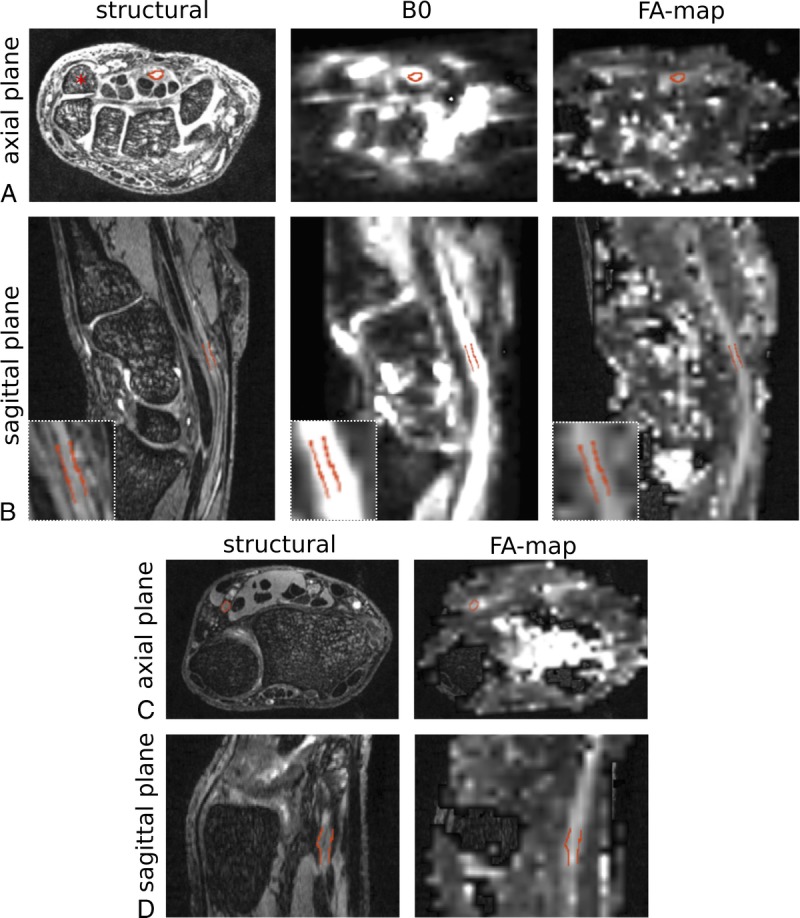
A and B, Registration of the unweighted (b = 0) images and fractional anisotropy (FA) maps to the morphologic images was successful at 7 T in the transaxial plane (A), which is commonly used for diagnostic purposes, as well as sagittal planes (B). The median nerve is delineated in red on the morphologic images at the level of the pisiform bone (asterisk). Registration reveals good alignment with the hyperintense median nerve structure on the unweighted b = 0 images. The median nerve is also clearly indicated by a region of elevated signal in the FA maps, with accurate registration to the morphologic image. Insets show magnifications of the outlined nerve. Because of the left-right phase encode direction, distortion artifacts are more apparent in the transaxial images (A) compared with the sagittal images (B). However, registration remains accurate. C and D, Fractional anisotropy (FA) maps of the ulnar nerve at the wrist are successfully depicted. Transaxial (C) and sagittal (D) sections of the wrist demonstrating successful registration of the ulnar nerve (red outline) on the T2-weighted image (left) with the FA map (right).
